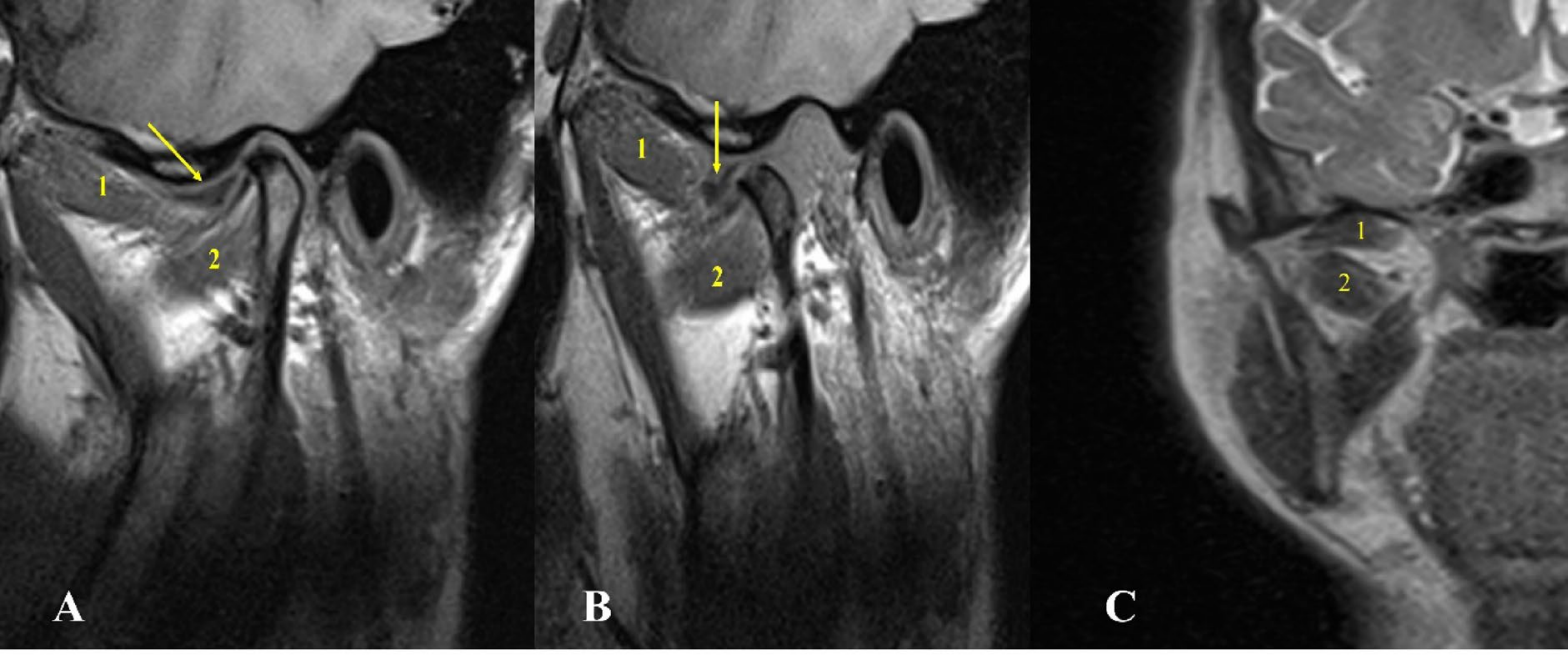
Oblique sagittal T1-weighted images represent Type II-A with two heads of lateral pterygoid muscle, the upper attached to the disc and the lower to the condyle associated with disc displacement without reduction and condylar erosion, osteophyte and bone marrow oedema.
